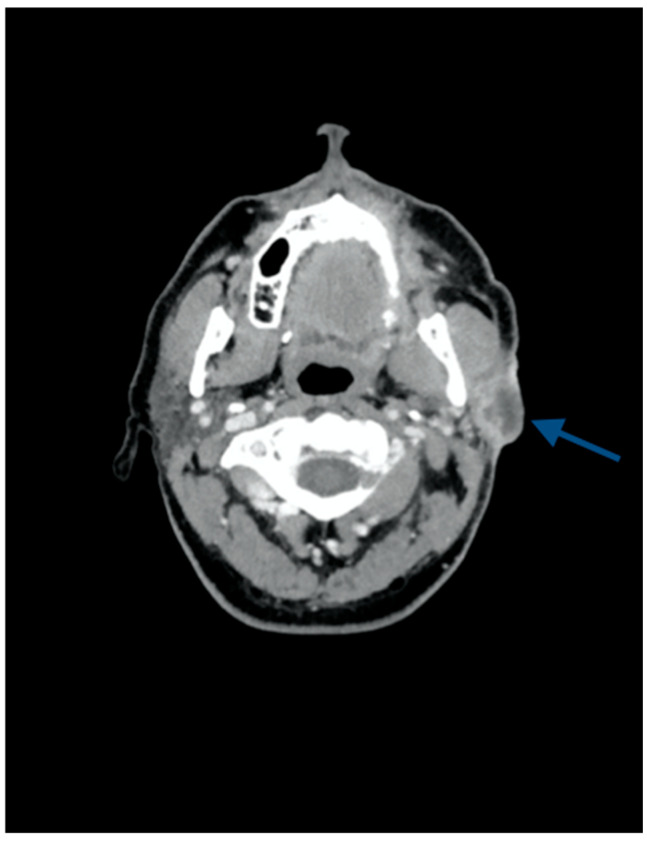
A CT scan displaying the skin neoplasm with deep invasion of the underlying parotid gland, the masseter muscle, and the anterior wall of the external auditory canal in its cartilaginous portion.
